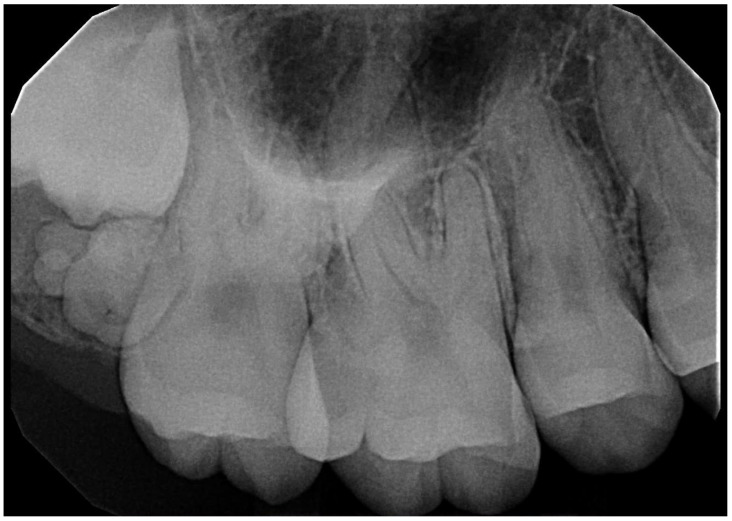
Periapical radiograph of a 20-year-old female patient showing a compound odontoma along the path of eruption of the third maxillary molar.
